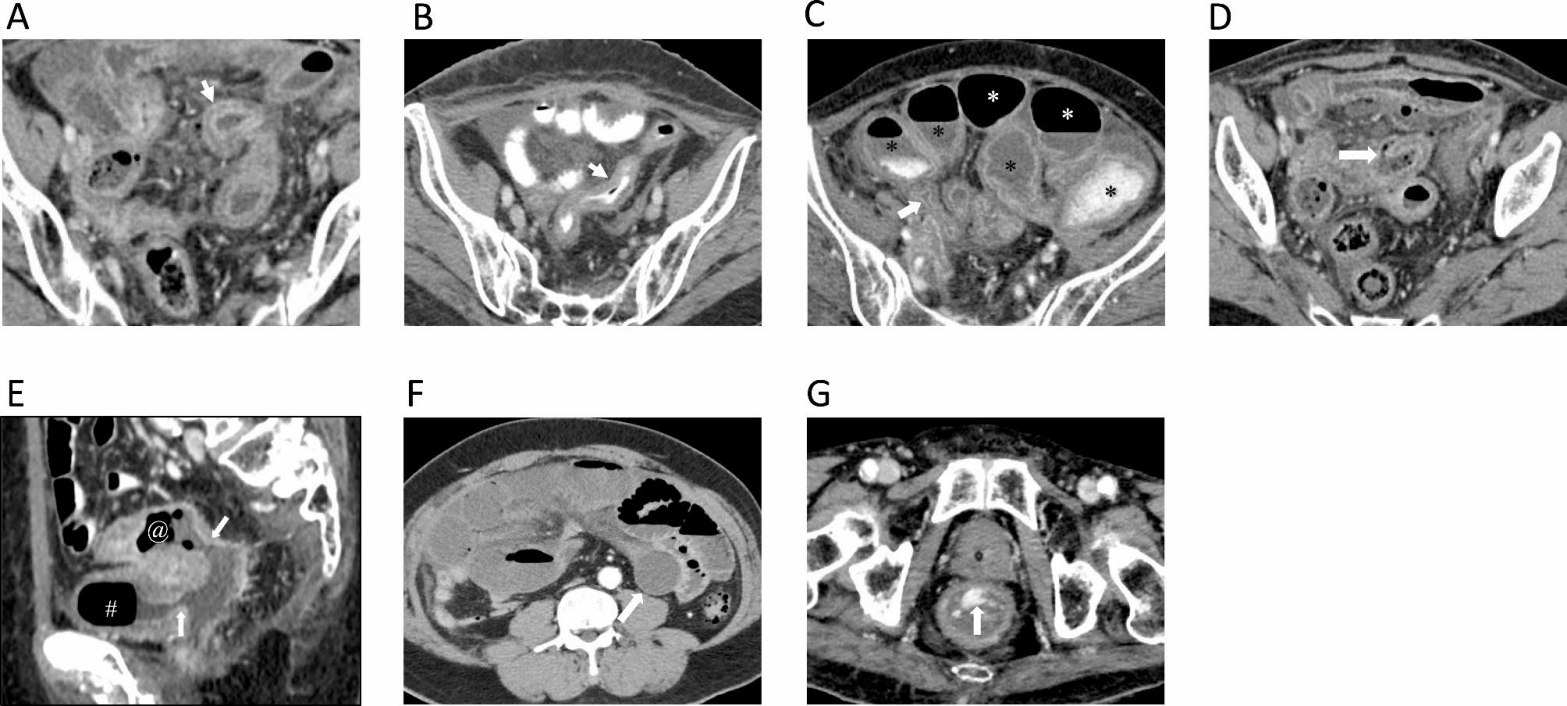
CT findings of radiation-induced late gastrointestinal toxicity. (A) Bowel wall thickening with target water bowel wall enhancement in distended bowel loop (arrow); (B) Bowel wall thickening with isoattenuation bowel wall enhancement in collapsed bowel loop (arrow); (C) Bowel obstruction; dilatation of the bowel loops [*] with transitional point (arrow); (D) Bowel wall disruption (arrow) in bowel perforation; (E) Sagittal CT shows fistula formation (arrow), connection between small bowel [@] and urinary bladder [#]; (F) Axial CT shows non-enhancing bowel wall (arrow) suggestive of intermediate to late-stage bowel ischemia. (G) Axial CT shows contrast extravasation into the rectal lumen (arrow)
